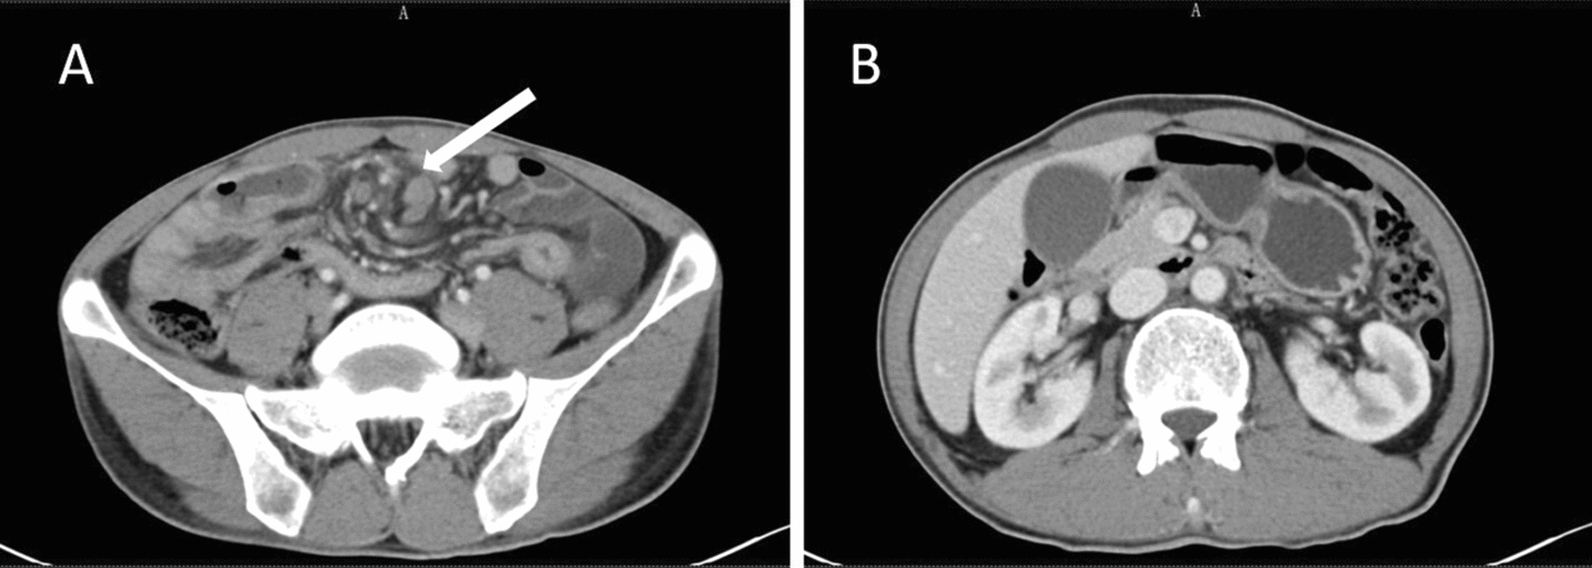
Abdominal enhanced computed tomography: A ileocecal wall localized thickening with persistent mucosal enhancement and surrounding mesenteric vessels showing a “whirlpool sign”; B progressive dilation of the intestinal lumen suggesting chronic partial bowel obstruction
